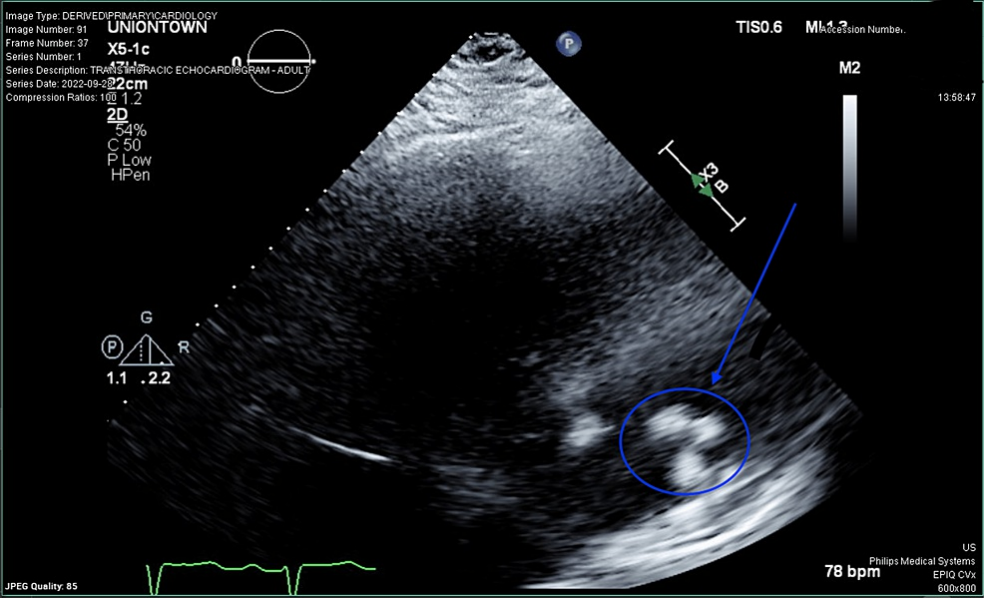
A right atrial mass was incidentally noted on initial transthoracic echography.
